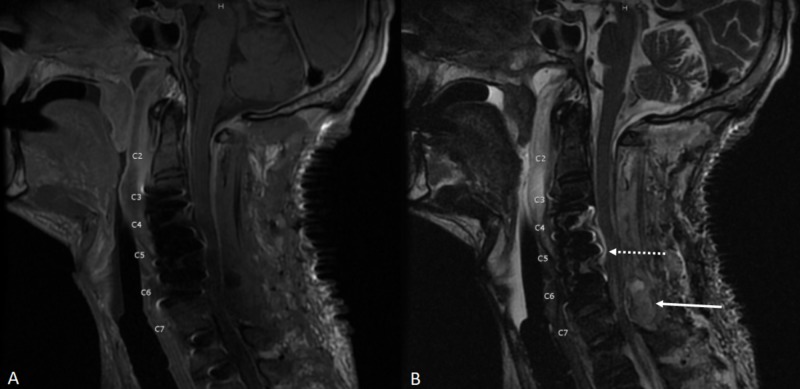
Sagittal MRI of the cervical spine (A) T1: Susceptibility artifact from the spinal fusion hardware limits evaluation and  (B) T2: Despite decompression, there is spinal canal stenosis with persistent mass effect and mild cord compression C4-5 (dashed arrow) and posterior cervical hematoma causing severe spinal canal stenosis and cord compression at C6-7 (solid arrow).
